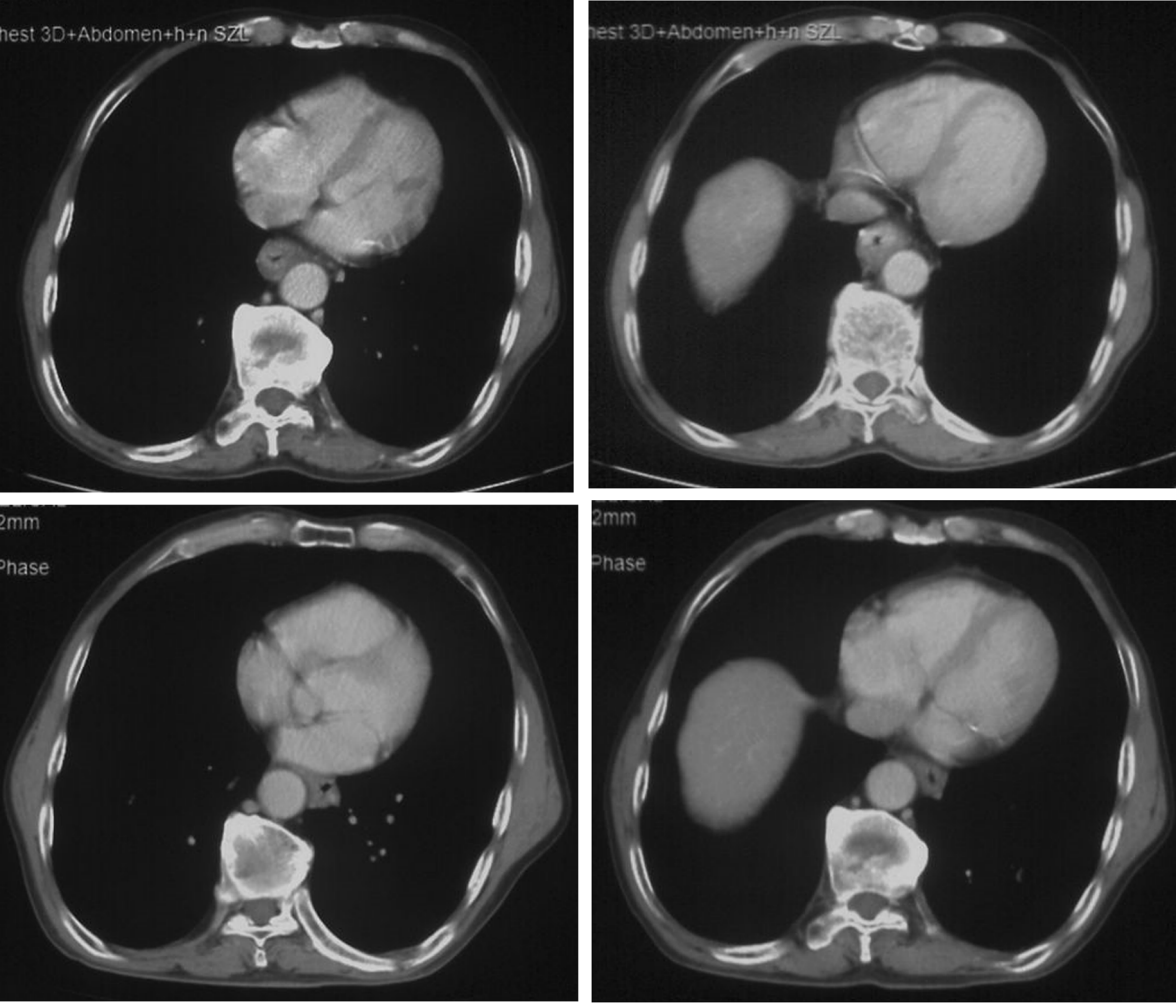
The CT images showed that esophagus had a dramatic radial escape after 2 cycles of chemotherapy and immunotherapy (lower) compared with the images before treatment (upper).
